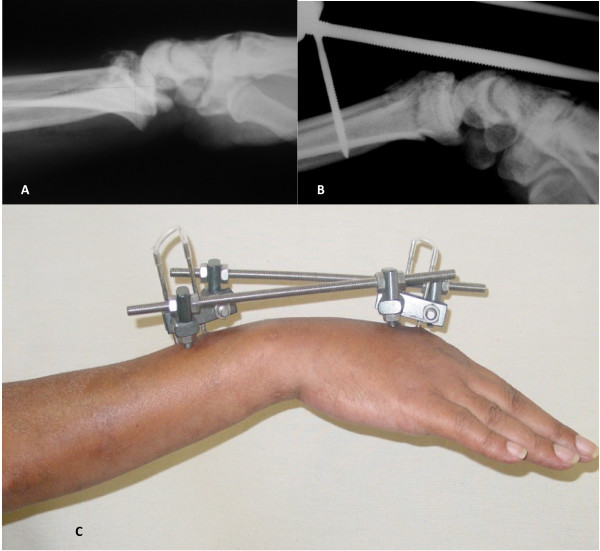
Bridging external fixation technique. A. Female, 50 years old. Radiograph preoperative in lateral view. B and 2C. The external fixator was positioned at an angle of approximately 90 degrees to the coronal plane of the forearm.
